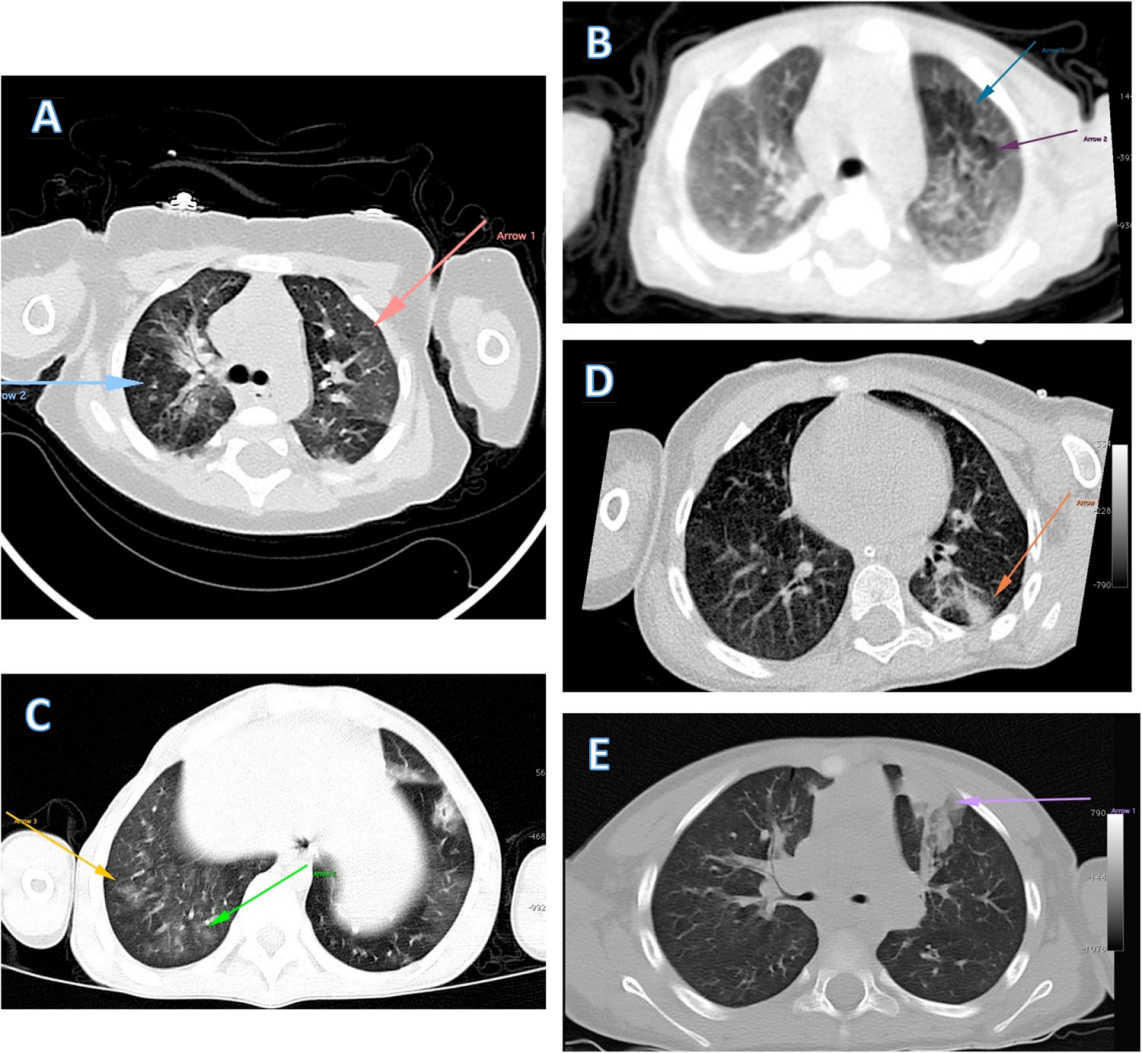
Key findings from different pediatric COVID‐19 patients with lung CT findings. In the first row, images (A) and (B) display prominent air trapping, particularly in image (B), suggesting severe underlying bronchiolitis. In image (C), we observe early stages of ground‐glass opacity in the right lower lobe of the right lung. Images (D) and (E) respectively depict early and significant consolidation in two different patients with COVID‐19 infection.
